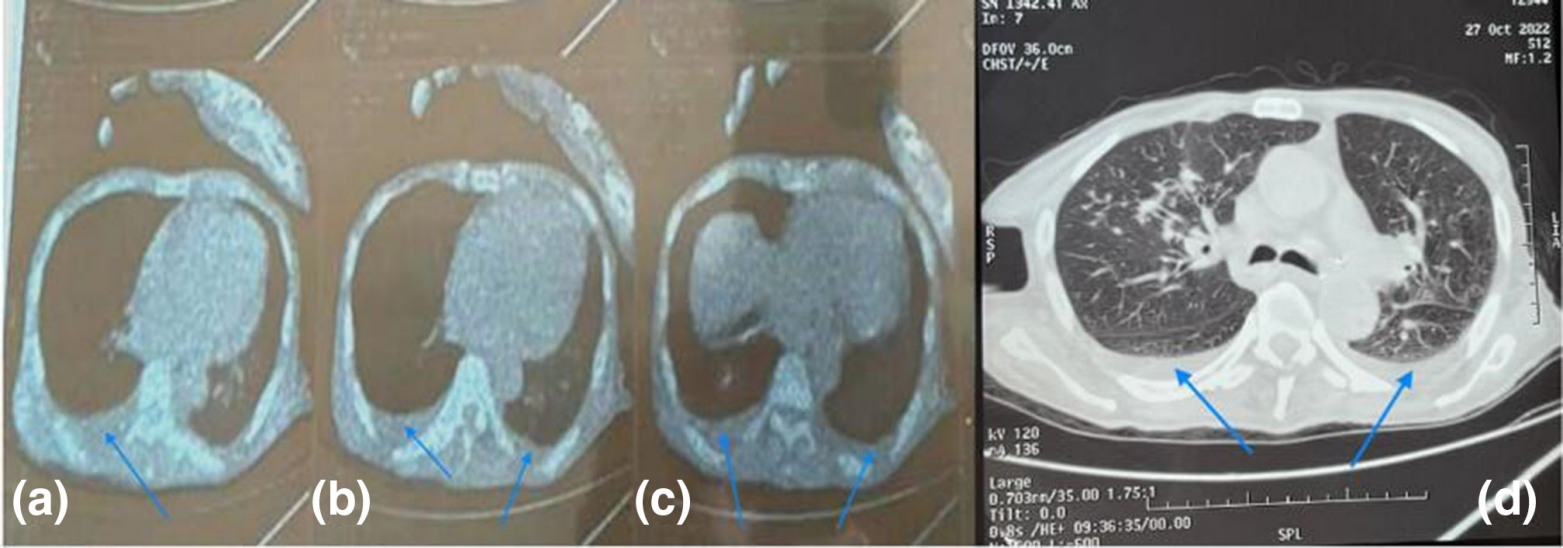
Axial CT scan mediastinal (a, b and c) and lung (d) windows showing bilateral pleural effusion (blue arrows) which is more pronounced on the right side.
